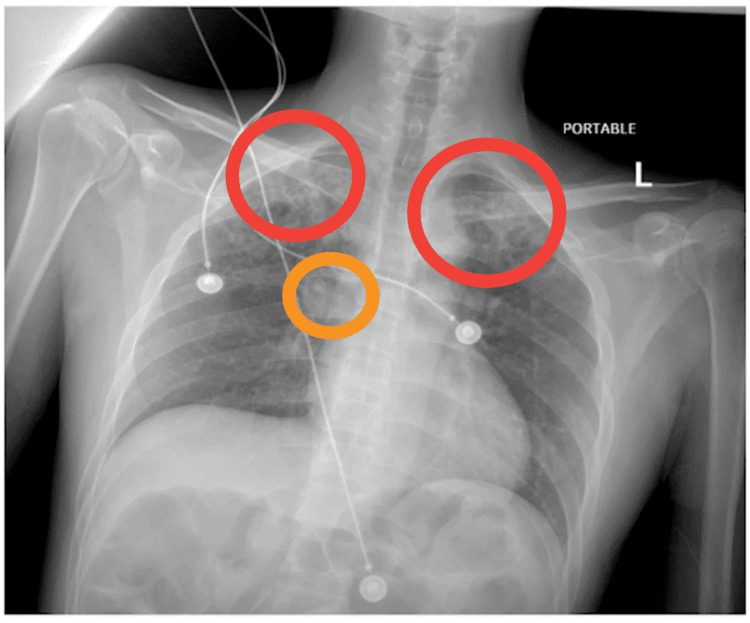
Chest radiograph demonstrating bilateral upper-lobe opacities with associated right hilar lymphadenopathy measuring approximately 2 cm Red: Bilateral upper-lobe opacities. Orange: Right hilar lymphadenopathy measuring approximately 2 cm
